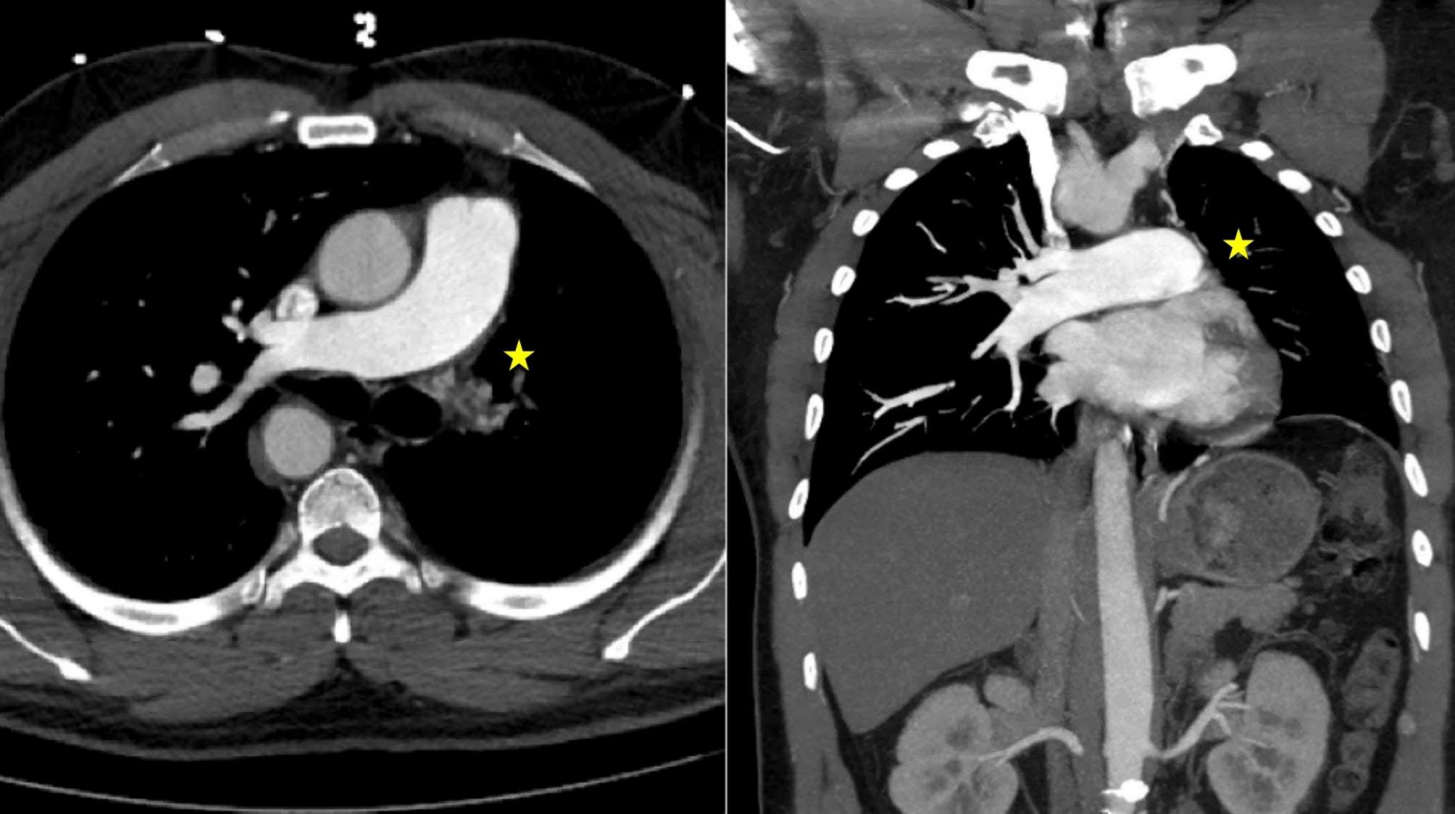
Computed tomography of an absent left pulmonary artery (identified with a yellow star)
